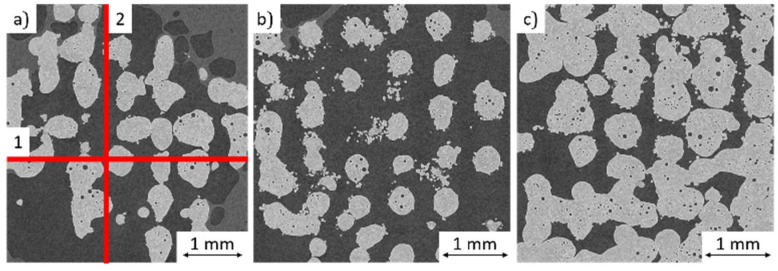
CT Image: (a) top view, (b) plane 1 sectional side view, (c) plane 2 sectional side view of a spherical structure (P_L_ = 300 W, v_s_ = 300 mm/s, g_w_ = 0.3 mm, l_s_ = 350 µm).
